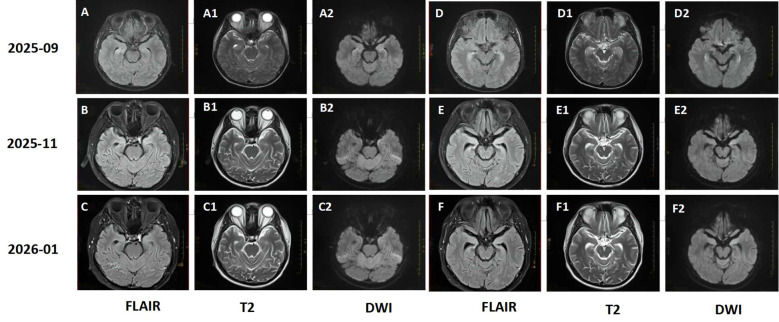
Brain MRI at onset showing abnormal signals in the bilateral hippocampi (A, D). Brain MRI demonstrating bilateral hippocampal alterations with enhancement on FLAIR (A, D), T2 (A1, D1), and DWI (A2, D2).Similar lesions exhibited recovery following 3 and 5 months of treatment. (B, B1, B2, E, E1, E2, C, C1, C2, F, F1, F2).
